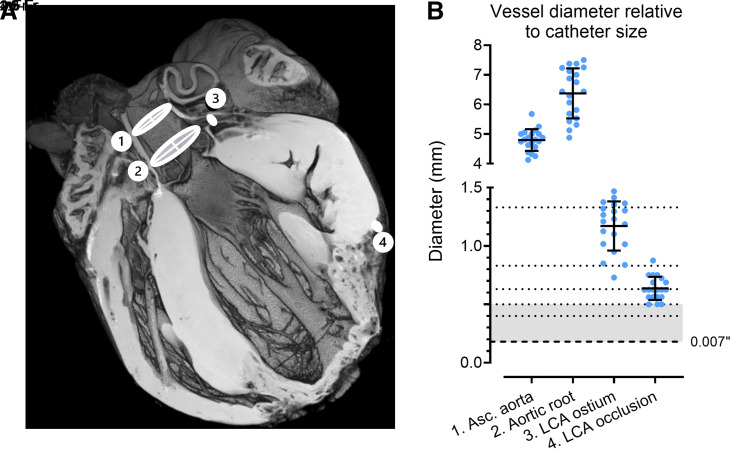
Vessel diameter relative to various catheter sizes. *A*: example T1w MRI image of a heart that underwent percutaneous occlusion. *1*) Axial cross-section includes ascending aorta, *2*) aortic root, *3*) left coronary artery (LCA) ostium, and *4*) LCA occlusion site in the apical artery. *B*: quantification of vessel diameters of hearts (*n =* 20) that were either used for ligation or percutaneous myocardial infarction (MI) procedures. Dotted lines represent relative sizes of angiographic- and microcatheters, and dashed line indicates the micro-guidewire. Gray zone represents the range of catheters and wires used in this study. Vessel diameter was measured just proximal to No. 4. Data are shown as means ± SD.
